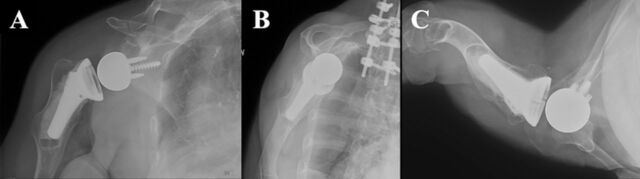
(A) Anteroposterior, (B) lateral, and (C) Velpeau view postoperative x-rays of the right shoulder demonstrate satisfactory alignment and orientation of reverse total shoulder arthroplasty.
